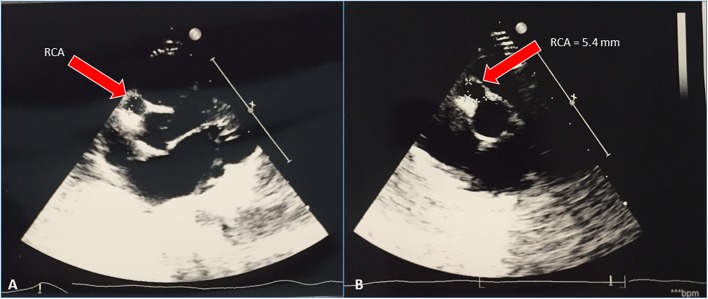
Heart ultrasound showing right coronary artery aneurism (RCA).
